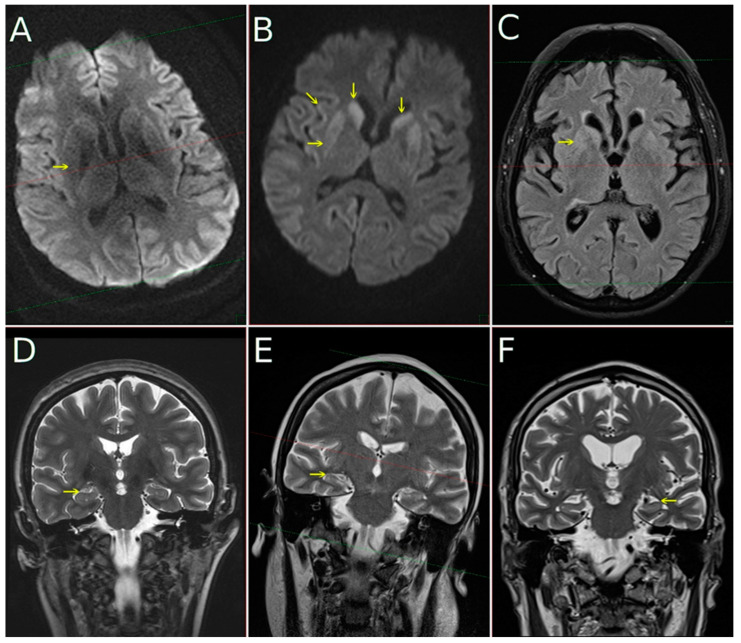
MRI in CJD subjects performed at 1.5T field strength: diffusion-weighed images ((A,B), DWI) with b-factor 1000, FLAIR image (C), coronal T2-weighted images (D–F). In the first column (A,D) data from subject with pure CJD—no DWI hyperintensity in putamina (arrow) is present and MTA is 0 (read as normal). In the second column (B,E) subject with tau comorbidity with mild hippocampal atrophy (MTA 1, arrow on (E)) and DWI (B) hyperintensity in putamina (horizontal arrow), caudates (vertical arrows) and with cortical ribboning (oblique arrow). In the third column (C,F) subject with CJD and AD comorbidity is shown. A moderate hyperintensity is visible not only on DWI (not shown), but as well on FLAIR image (C). Hippocampal atrophy is well pronounced, MTA 2 (arrow from right side of the (F), pointing to the left hippocampus which manifests clear atrophy).
